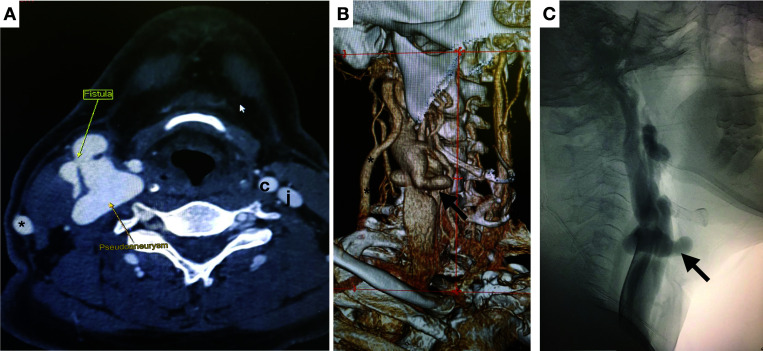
Preoperative examinations. A) Computed tomography of the neck (axial slice); B) volumetric rendering; C) selective arteriography of the right common carotid (lateral view). Smaller arrow: arteriovenous fistula; larger arrow: carotid pseudoaneurysm; * right external jugular; c: left common carotid, j: left internal jugular.
